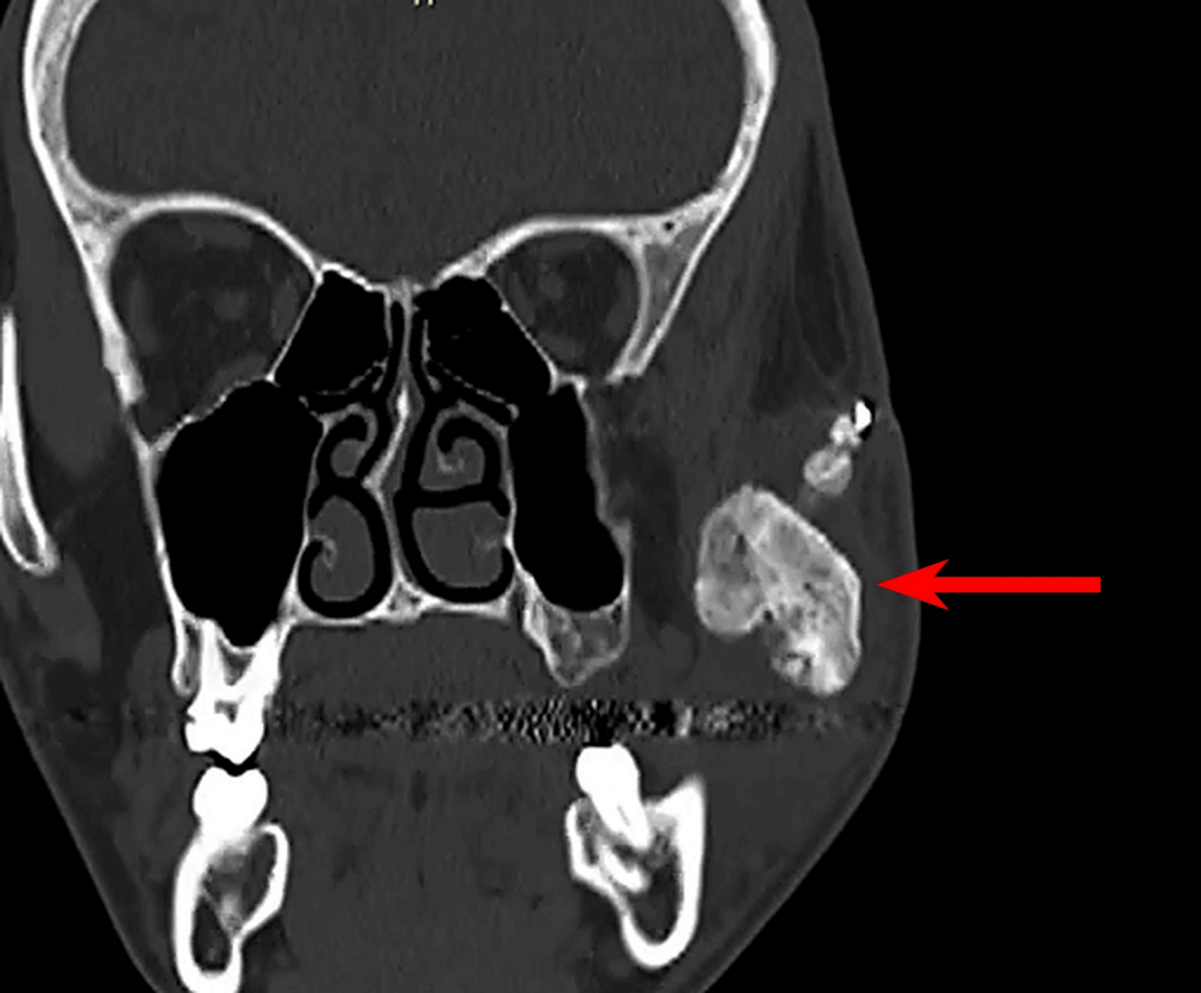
Coronal CT (bone window) showing heterotopic ossification in the left masseter muscle (red arrow).
